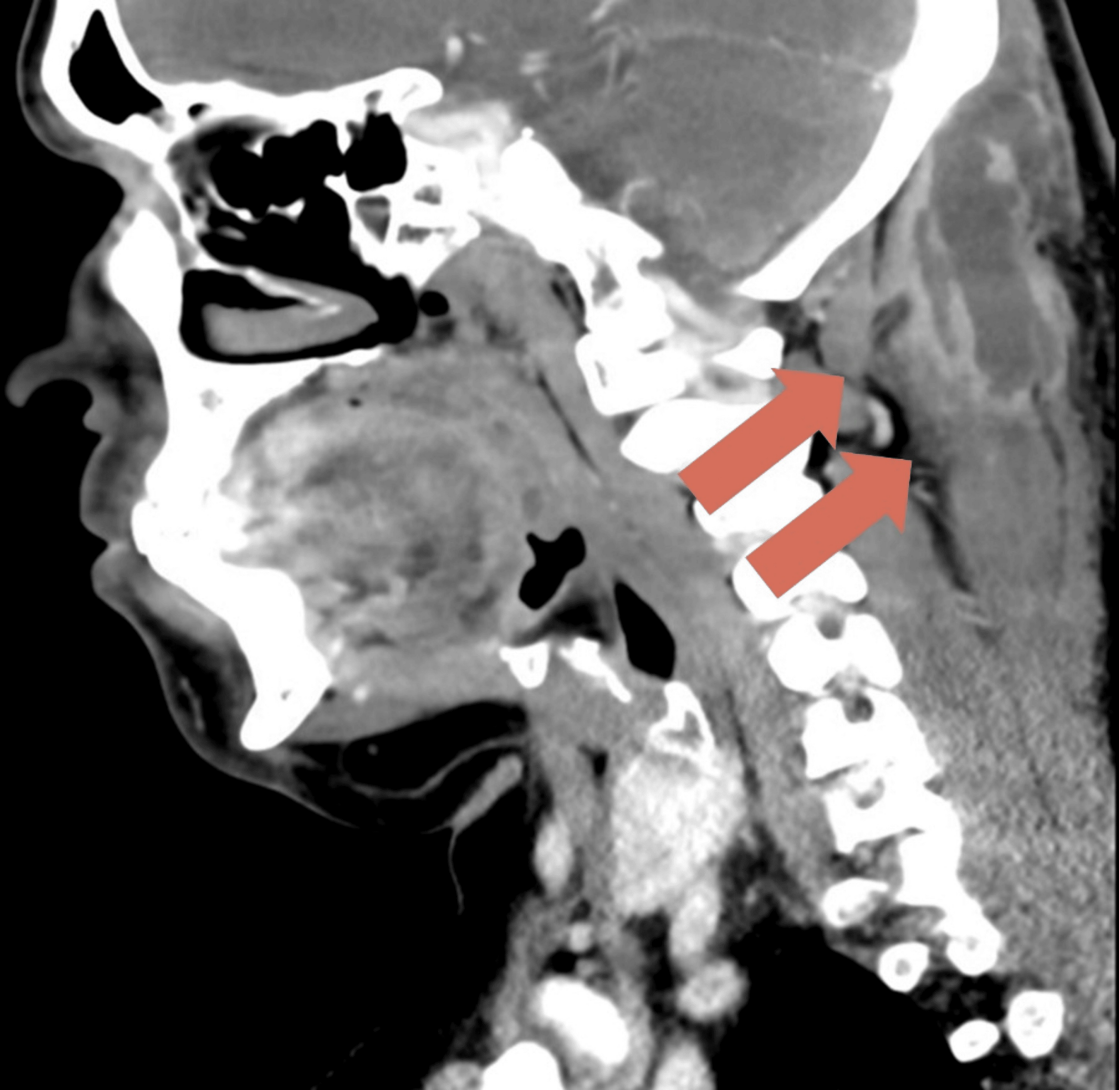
Contrast-enhanced CT of the cervical spine demonstrating deep paraspinal abscess Contrast-enhanced CT scan of the cervical spine on hospital day 1 showing a rim-enhancing fluid collection measuring 6.6 × 3.5 × 1.6 cm. The abscess extends from the occipital region to the level of the C2 vertebra. No bony destruction or intracranial extension is identified.
